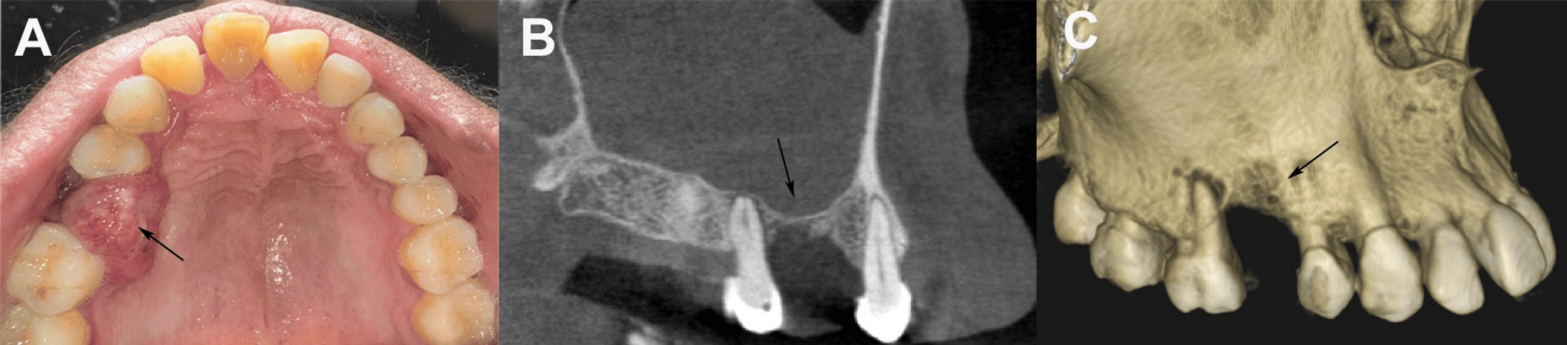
(A) Clinical photograph showing a 10×12×15 mm irregular, soft, pedunculated, exophytic, dome-shaped growth on the edentulous #15 alveolar ridge, with a red, inflamed, lobulated surface and erythematous surrounding mucosa. (B) Cone-beam computed tomography (CBCT) scan revealing erosion of the alveolar bone in the #15 region, approaching the maxillary sinus floor. (C) Additional CBCT view highlighting significant alveolar bone loss in the #15 region, raising concerns for potential oroantral communication. Patient images were obtained with informed consent for publication.
